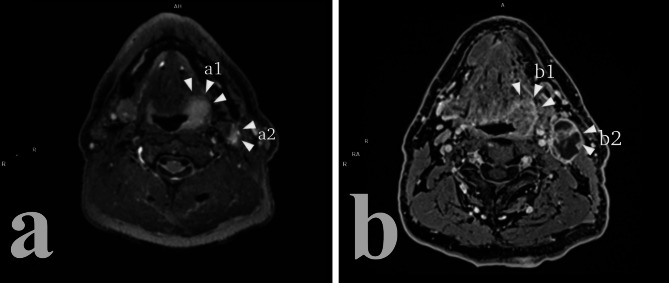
HPV + oropharyngeal cancer (a1, b1) with adjacent cystic metastatic lymph node (a2, b2). (a) T2w; (b) post-contrast T1w. The relative higher soft tissue resolution of MRI highlights the contained nature of the lesion and its well-defined borders
